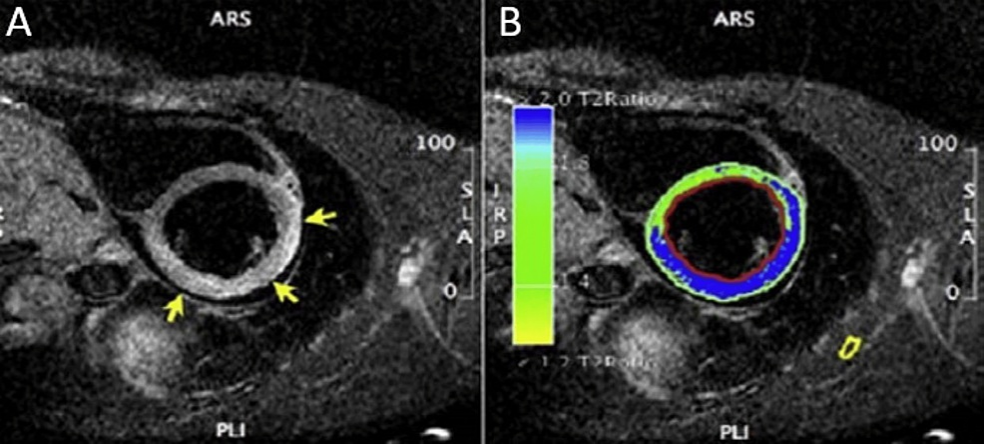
CMR examination of a 23-year-old male patient diagnosed with myocarditis-T2 images CMR: Cardiac magnetic resonance (A): T2-weighted CMR image showing subepicardial oedema in the inferolateral, inferior, and anterior segments. B: Computer-aided signal intensity analysis of the T2-weighted image with color-coded display of relative signal intensity, normalized to skeletal muscle. Blue indicates a signal intensity ratio of myocardium/skeletal muscle of ≥2.0, indicating oedema, and green indicates normal signal intensity. Image courtesy: Personal archive of Dr. Oana Popa. Patient consent was obtained.
